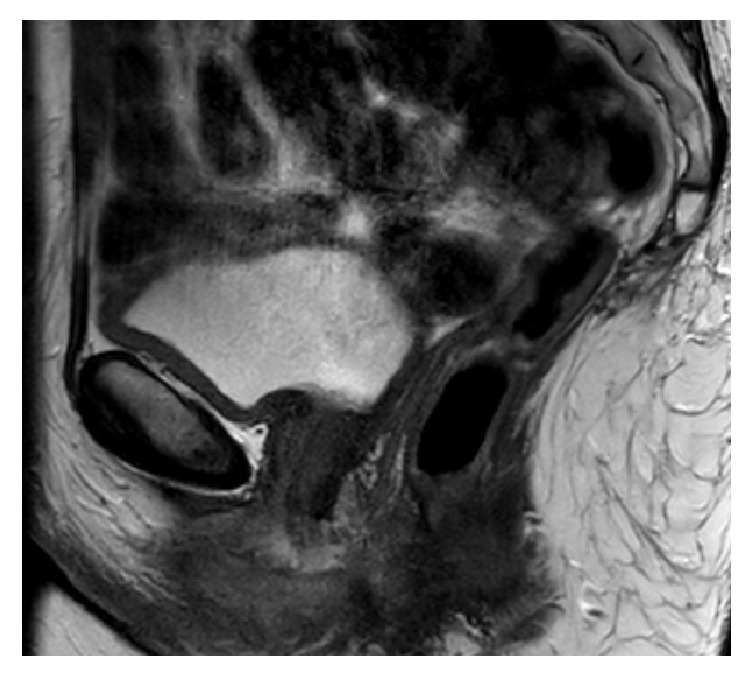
MRI of the female pelvis.* The urethra is clearly visible without the presence of a urethral diverticulum or periurethral abscess.*
